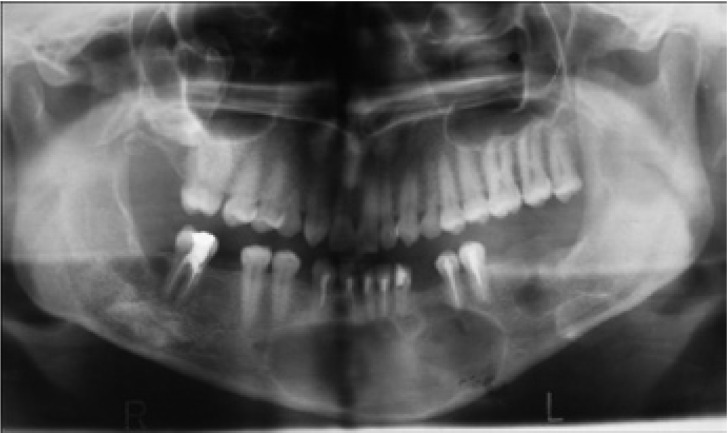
Panoramic radiograph of a KCOT occupying mandibular symphsis, between the right and left second premolars. Note the root resorptions of the anterior mandibular incisors. Slight appearance of the radiolucent areas in the right and left ramus, and in the posterior part of the right mandible associating with the second molar.
